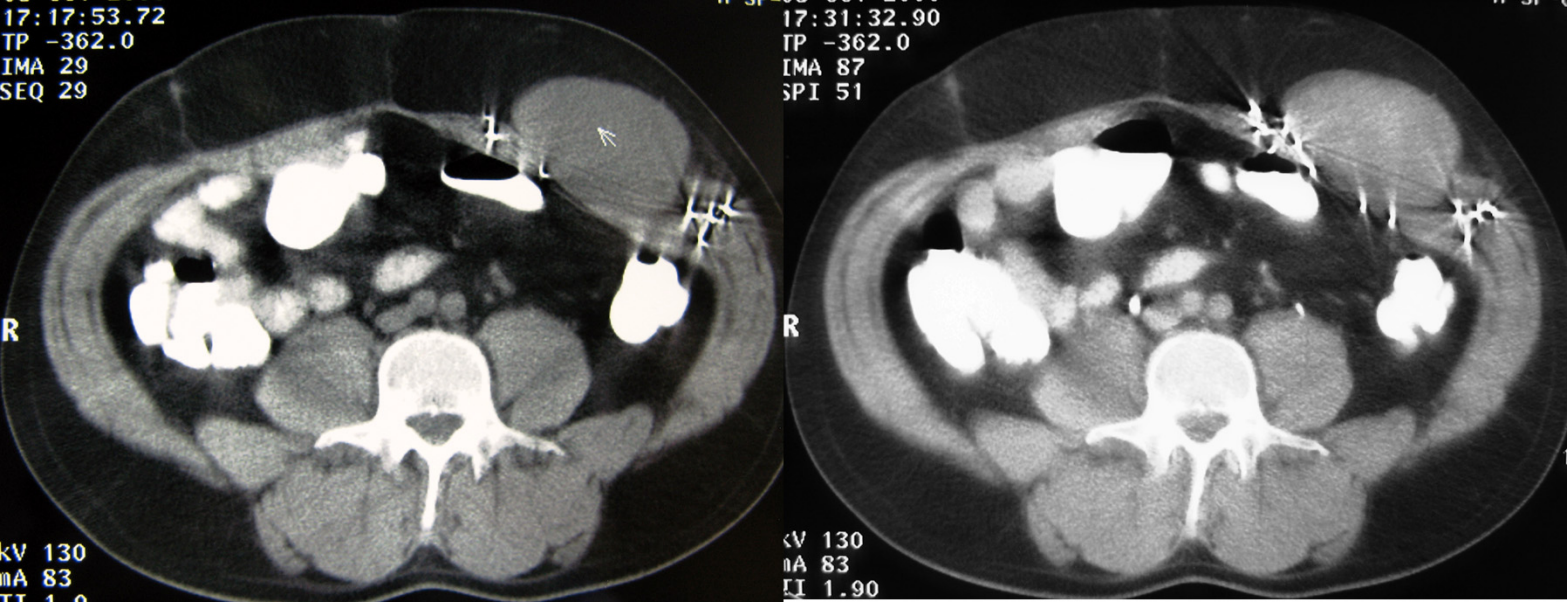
Axial CT scan images of the abdomen. Computed tomography examination before (A) the intravenous administration of contrast medium revealing a well-circumscribed mass originating from the left rectus abdominis muscle and of attenuation equal to that of the muscle. The mass demonstrates a mild enhancement even in the delayed images after the intravenous administration of contrast medium (B). The presence of multiple foreign bodies caused by the shotgun injury.
